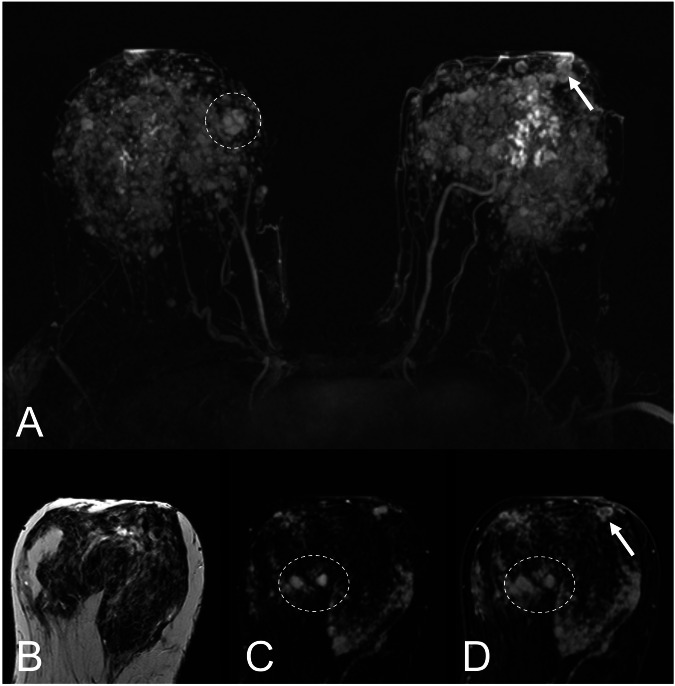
34-year-old patient with dense breasts (ACR D) and marked bilateral BPE. The lesion (arrow) is not well visible on the MIP (A) and T2w (B), while it can be seen well on early (C) and late (D) contrast-enhanced subtractions. The lesion presents as an oval rather circumscribed mass with central Wash-out, corresponding to a Kaiser score of 4 (low level of suspicion). Discharge could be provoked, and associated ducts were visible (not shown). Diagnosis was BI-RADS 4a (suspected papilloma) with targeted ultrasound-guided biopsy revealing papilloma without atypia. Note that several benign lesions are visible (dashed circle), partly also on the MIP (A)
